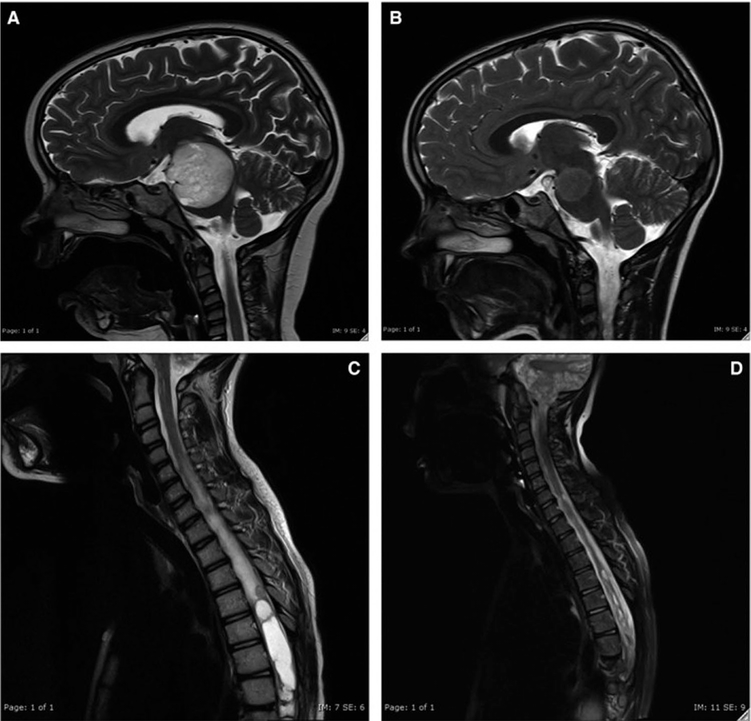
Radiological response to bevacizumab therapy; MRI imaging at baseline PLGG (A) and PLGG10 (C) and 15 mo after completion of therapy PLGG8 (B) and after 6 mo of therapy PLGG10 (D) (Zhukova *et al*., 2019).
